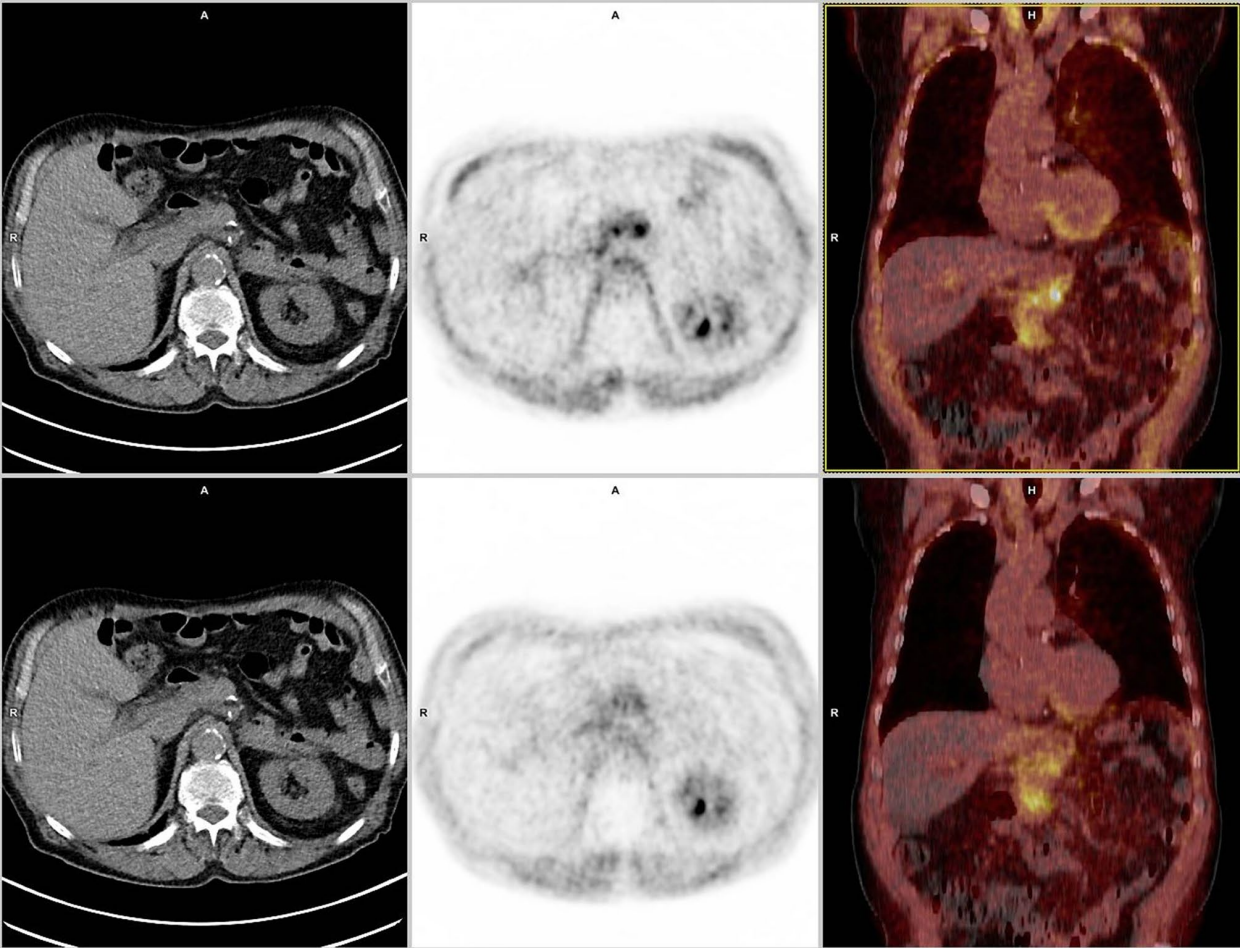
Showing patient J with a recurrent pancreatic ductal adenocarcinoma at timepoint *t*_*1*_ (upper row) (timepoint *t*_*1*_ SUV_max_ 8 and MTV 63 cm^3^) and posttreatment images at timepoint *t*_*2*_ (lower row) (78 days after radiation therapy treatment completion; timepoint *t*_*2*_ SUV_max_ 4 and MTV 29 cm^3^). Upper and lower left-side: unenhanced CT; upper middle column: pretreatment [^68^Ga]FAPI-PET; lower middle column: posttreatment [^68^Ga]FAPI-PET (both axial reconstructions at the level of the liver and left kidney); upper and lower right-side: fused mode [^68^Ga]FAPI-PET/CT at timepoint *t*_*1*_ (upper row) and [^68^Ga]FAPI-PET/CT at timepoint *t*_*2*_ (lower row) [point-spread function (PSF) reconstruction; PET Heat 2D, 13 min after 52 MBq [^68^Ga]FAPI-injection at timepoint *t*_*1*_ and 16 min after 95 MBq [^68^Ga]FAPI-injection at timepoint *t*_*2*_]. Focal pathological uptake on follow-up PET-images considerably declined.
